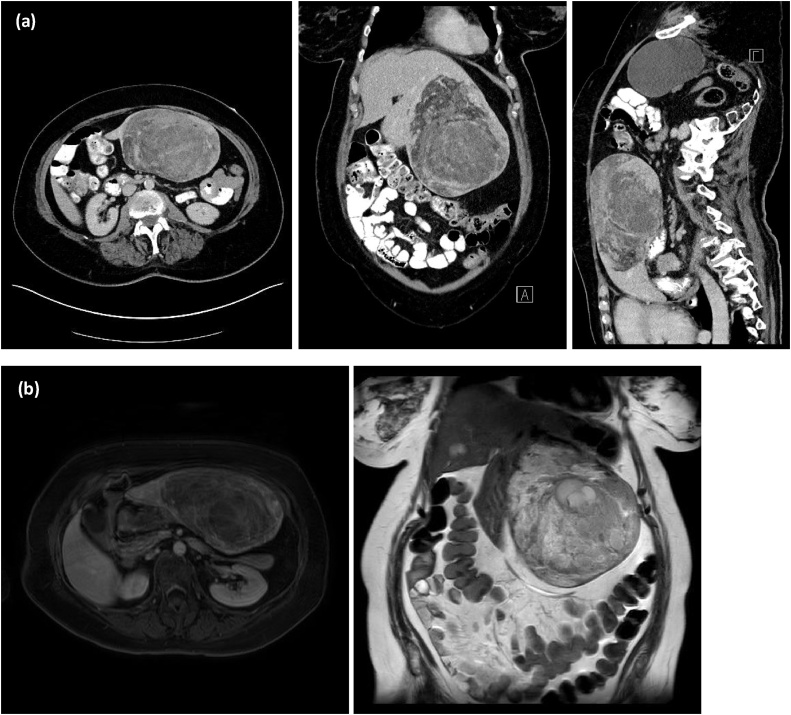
(a) Contrast-enhanced CT showed a 17 cm in diameter tumour in the left lobe of the liver. (b) MRI showed a 1250 mL volume mass with inhomogeneous intensity in T1- and T2-weighted sequences in left liver segments II and III with cystic and haemorrhagic components, a marginal hyperperfusion and diffusion restriction, and large fatty areas.
